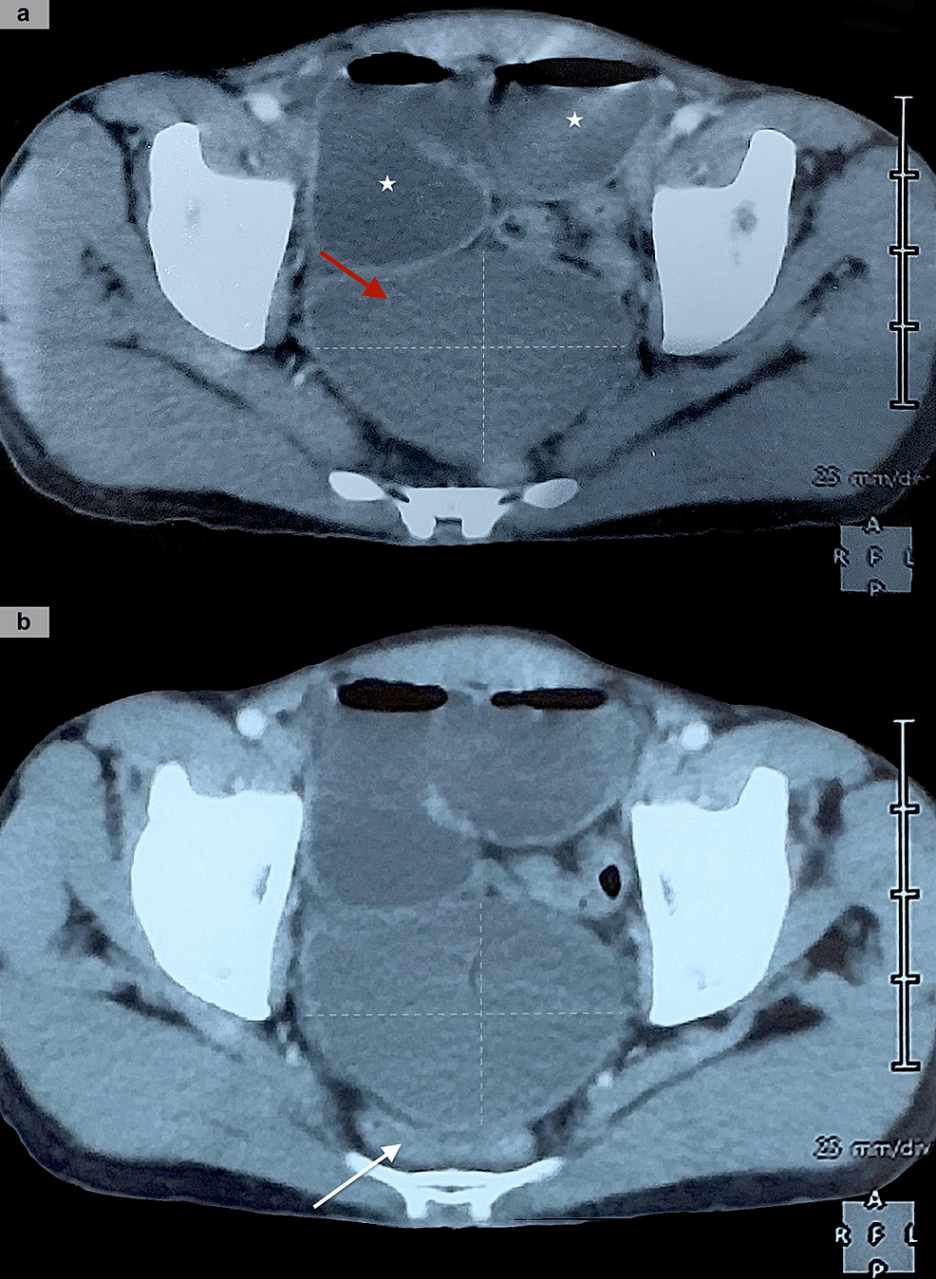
Axial CT scan revealed a well-circumscribed cystic mass (red arrow), located in the pelvis, along with numerous gas-fluid levels in distended bowel loops (white star) (a). The cyst caused a mass effect on the rectum (b).
